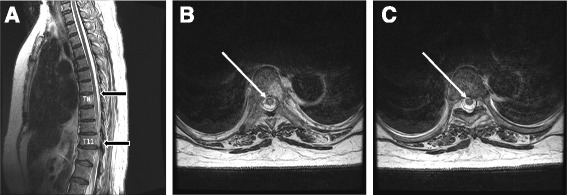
a Sagittal magnetic resonance imaging (MRI) revealed hyperintensity in the thoracic cord from T8 to T11 (*arrows*); spinal cord infarction was suspected. b Axial MRI of the T8 spinal cord level. A hyperintense area was apparent (*white arrow*). c Axial MRI of the T6 spinal cord level. Normal intensity was observed (*white arrow*)
